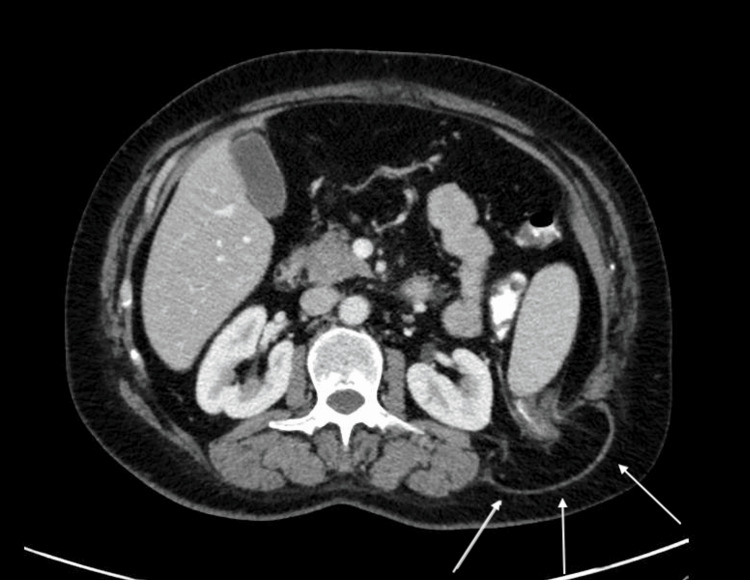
CT axial view. The arrows indicate the lumbar hernia defect.
